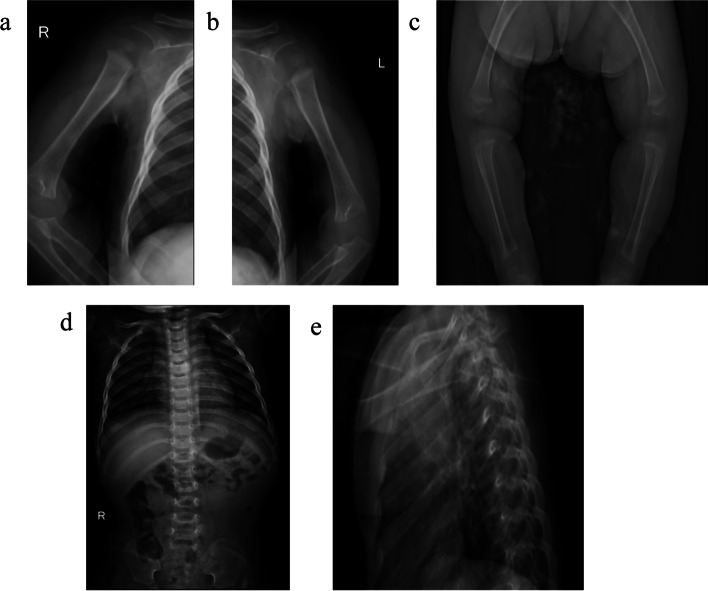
The findings on plain X-ray examination (a, b, c) Mild metaphyseal flaring in long bones, more evident in lower limbs, and short femoral necks. d, e spine front and lateral view. No narrowing of interpedicular distance between the first and the fifth lumbar vertebral bodies.
